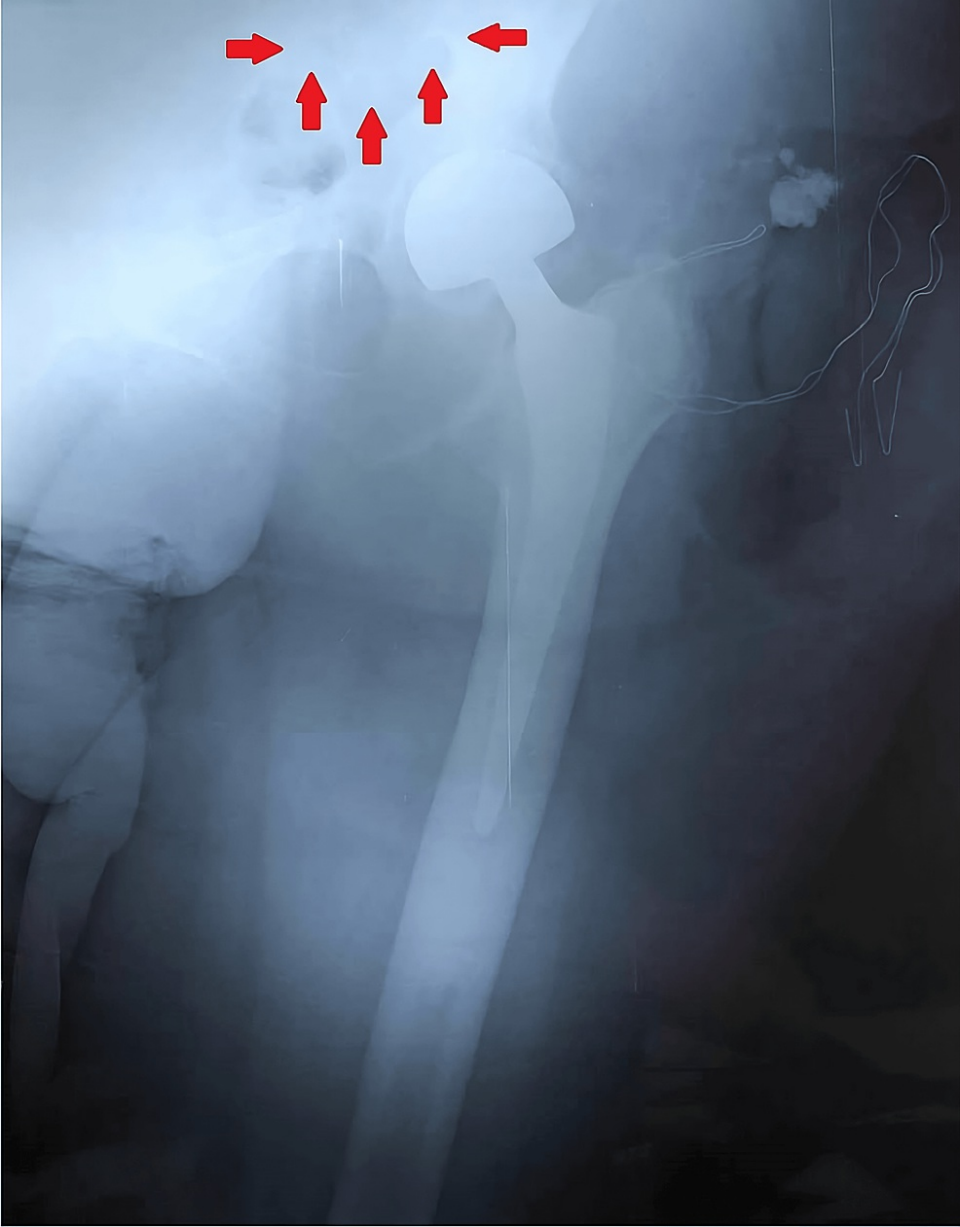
Intraoperative left hip radiograph. Left hip anteroposterior radiograph, after supine positioning of the patient, to facilitate the detection of the trial components, with no success due to their inherent radiolucency. The arrows mark the area where trial components were eventually retrieved (between the psoas muscle and the transverse abdominal muscle).
